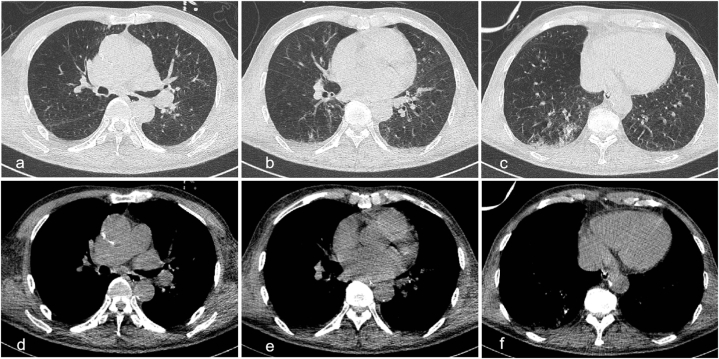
The chest CT scan on day 7 after ICU admission. (a–c) The lung window images. (d–f) The mediastinal window images. CT, computed tomography; ICU, intensive care unit.
